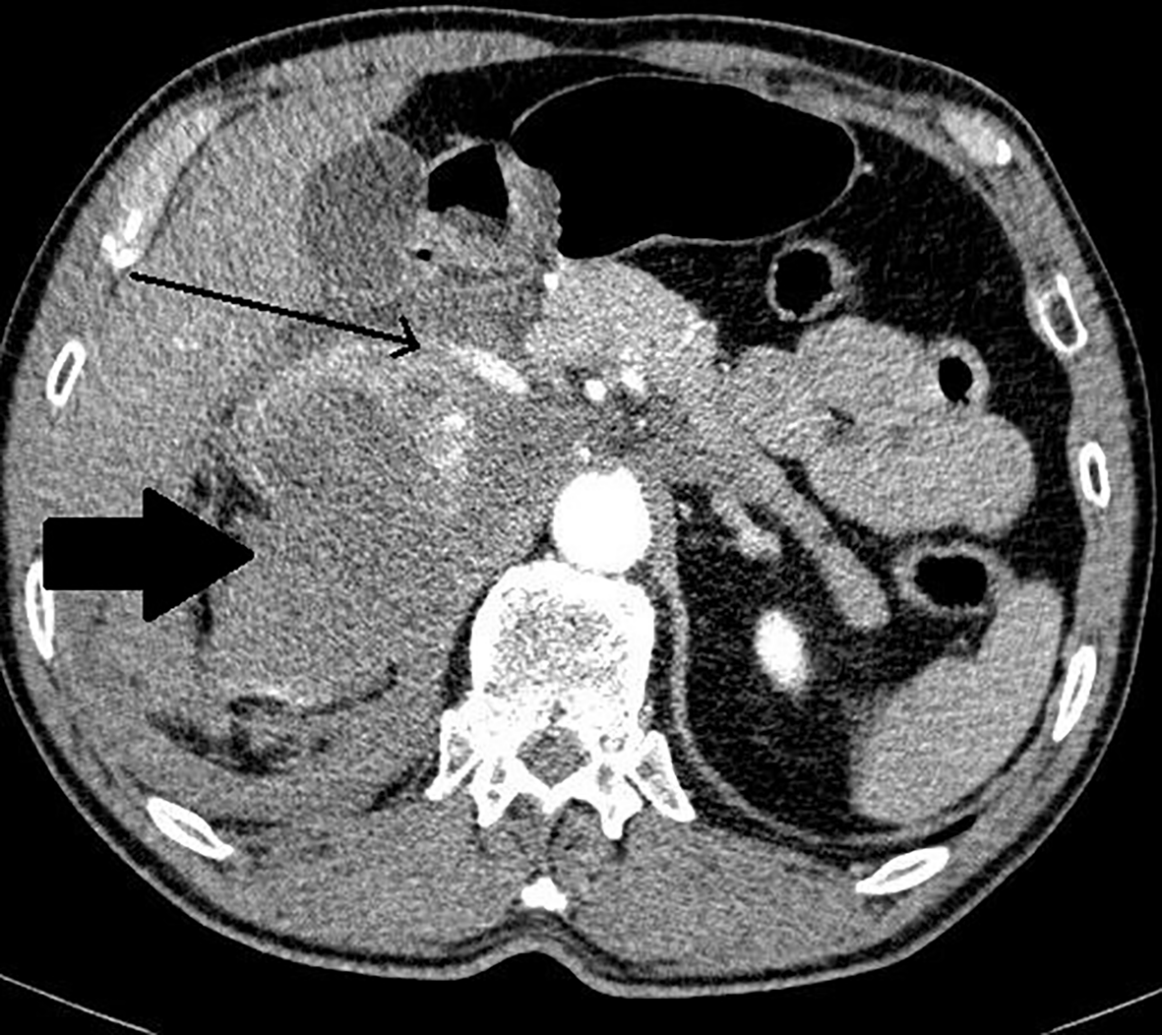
Patient number 2: angio-CT, arterial phase, axial image- right adrenal mass with heterogenous enhancement (thick arrow), suspicion of rupture of central part of the tumour with contrast extravasation (thin arrow). Right adrenal gland is not separately visualized. Left adrenal gland visible, with physiological contrast enhancement.
